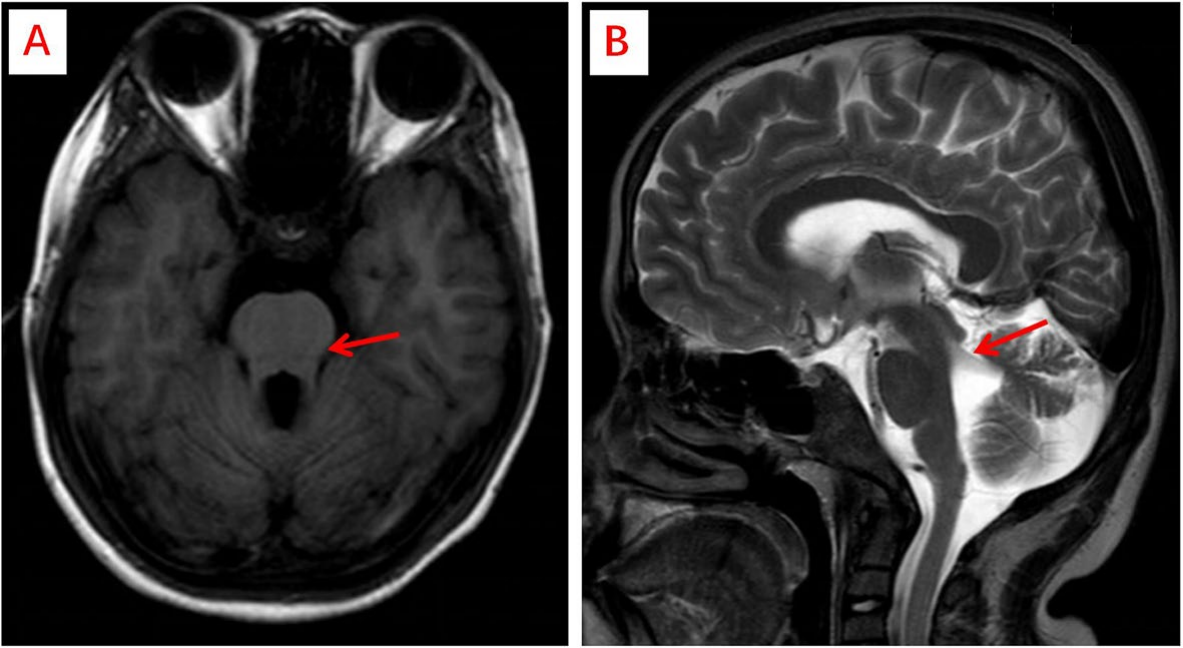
Brain MRI showed classic findings of Joubert syndrome. A shows extension of the upper cerebellar feet with the “molar tooth sign” (arrow); (B) shows a thickened upper cerebellar foot almost perpendicular to the brainstem (arrow)
